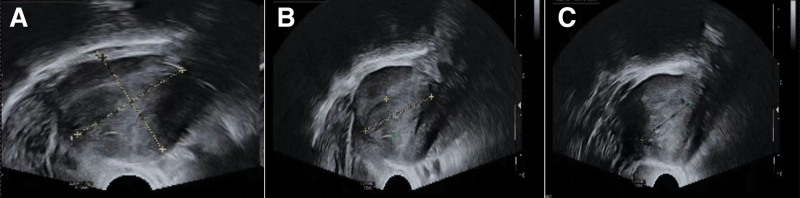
(A–C) Transvaginal ultrasound (TVS) examination shows that the uterus is slightly enlarged, and there is a lack of homogeneous echogenicity in the uterine cavity.
